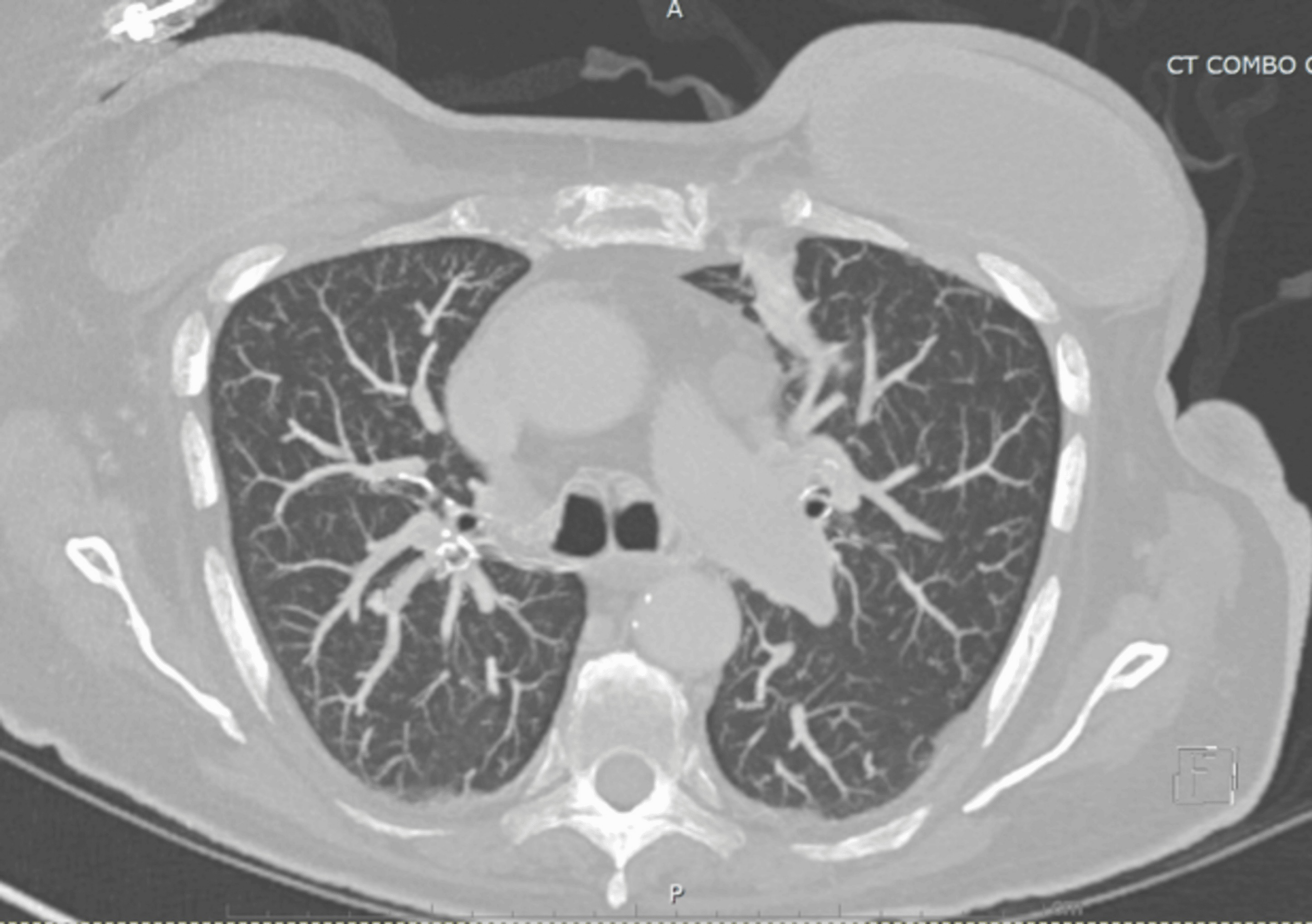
Chest CT scan showing marked improvement in consolidations six months later.
